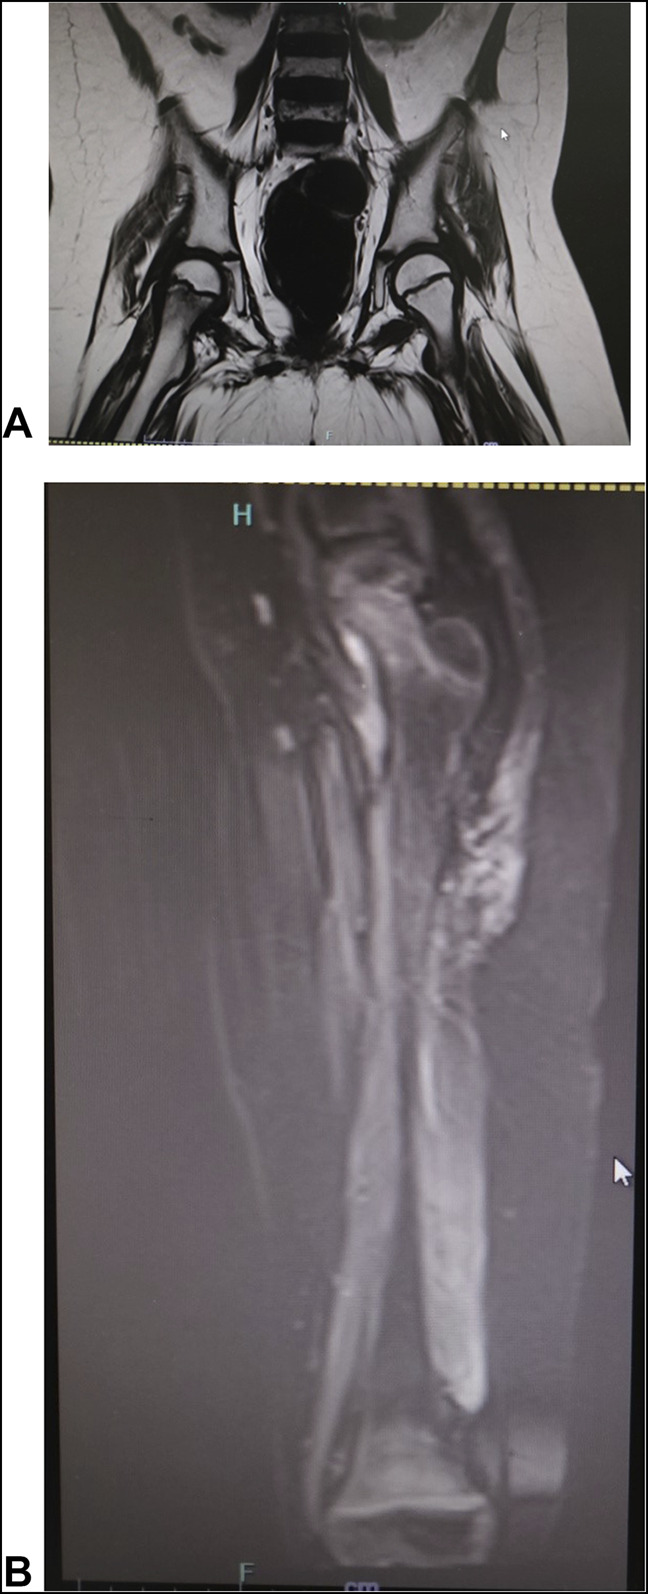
A, MRI of both hips showing diffuse marrow edema bilateral proximal femurs, more on the right side. Hip joints are normal. B, MRI of left thigh showing diffuse marrow edema along the femur. Thigh and gluteal muscles also show hyperintense signals.
